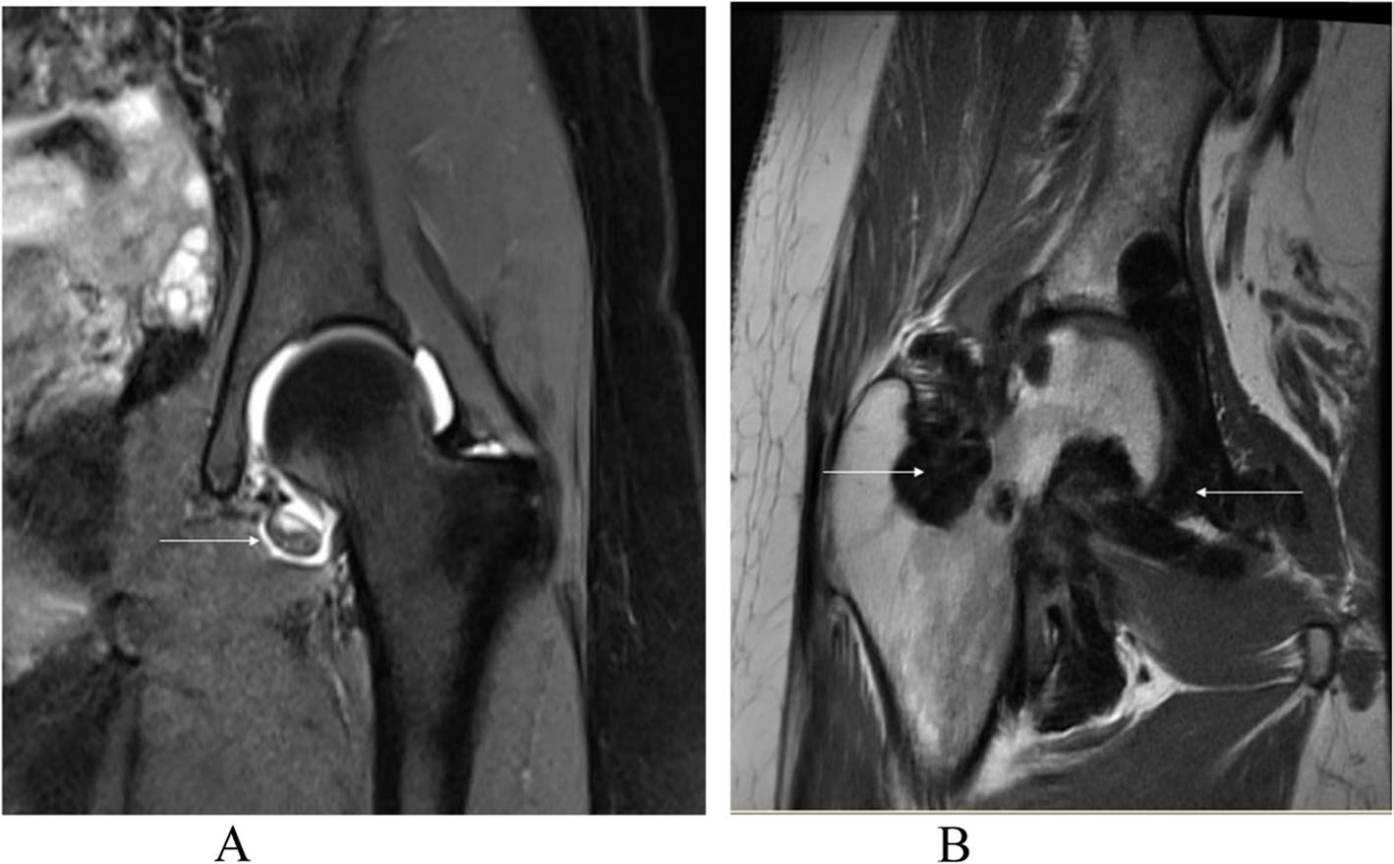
MRI findings of diffuse or localized type of PVNS. A: MRI coronary PD-weighted image of the left hip of localized PVNS, a wide band of short T2 signal nodule can be seen medial to the articular space. B: MRI coronary T1-weighted image of the right hip of diffuse PVNS, multiple long T1 mixed T2 signal shadows can be seen in the articular space. White arrows indicate the lesion sites
